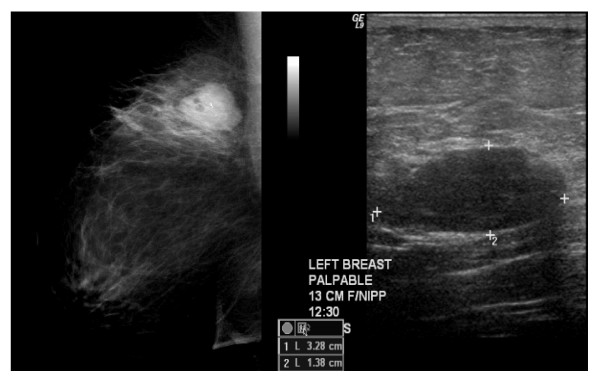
Breast Imaging. (A) Digital mammogram (B) ultrasound showing dominant mass in the upper central portion of the breast.
